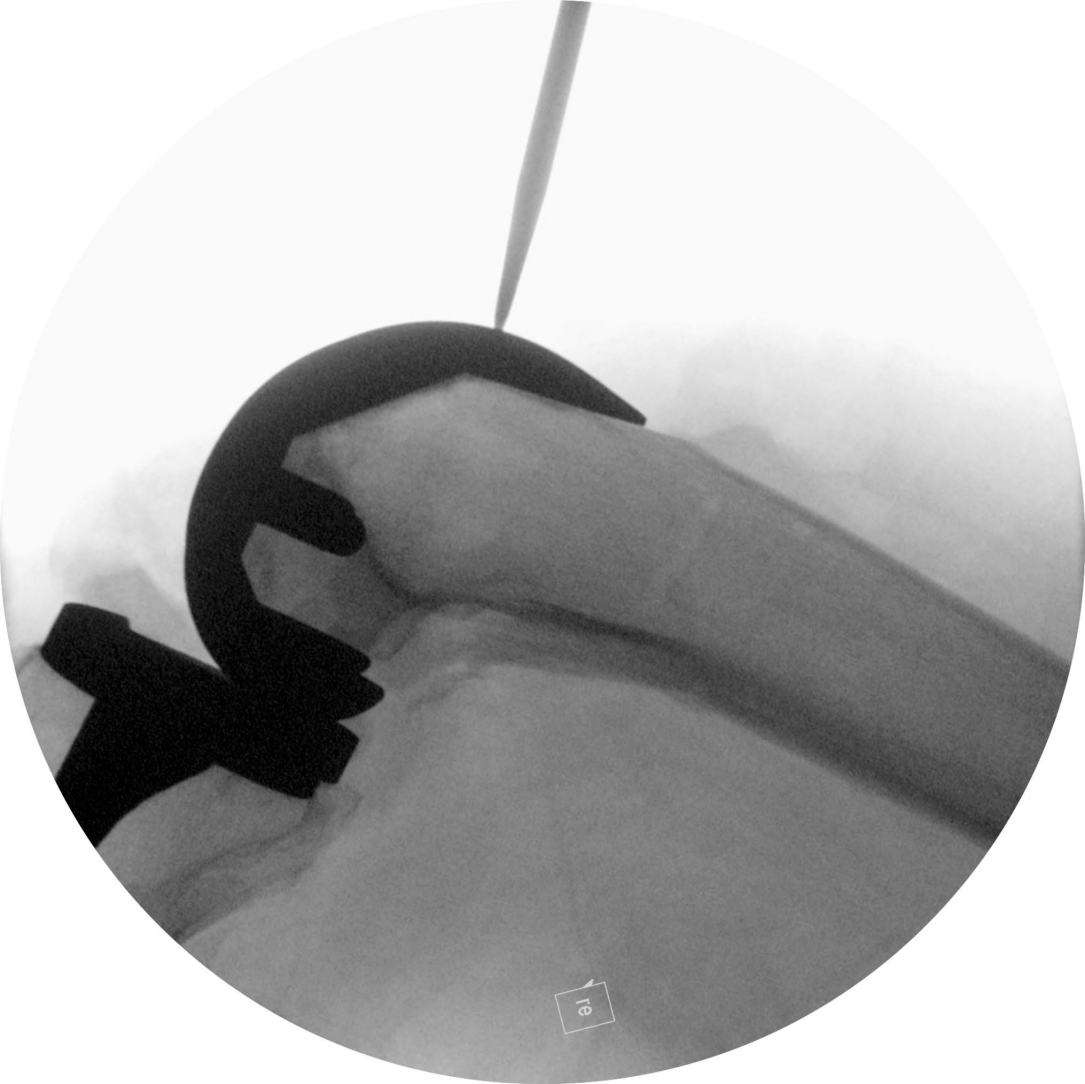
Lateral x-ray view demonstrating the sharp probe’s tip omitted by the lateral facette
